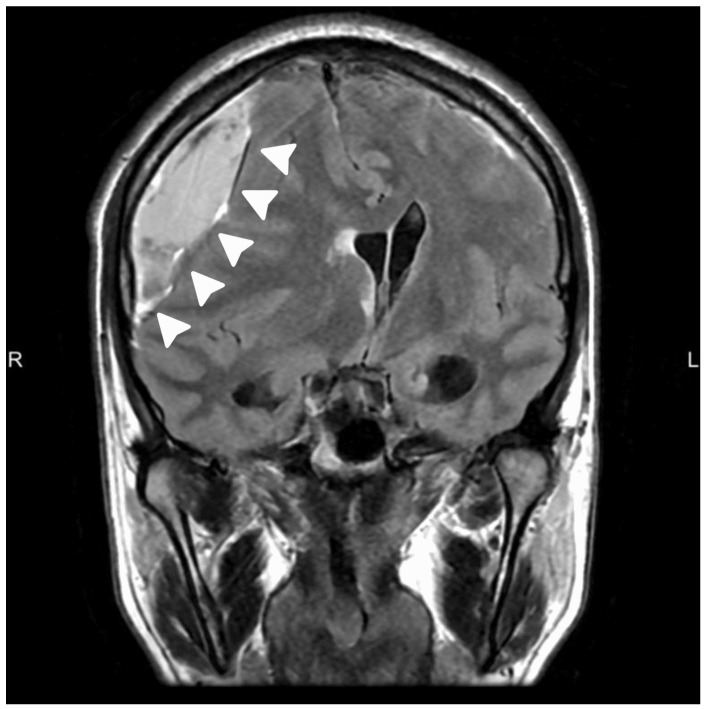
Pre-treatment coronal T2 FLAIR MRI showing a right-sided chronic subdural hematoma (arrow) with significant mass effect and subfalcine herniation.
